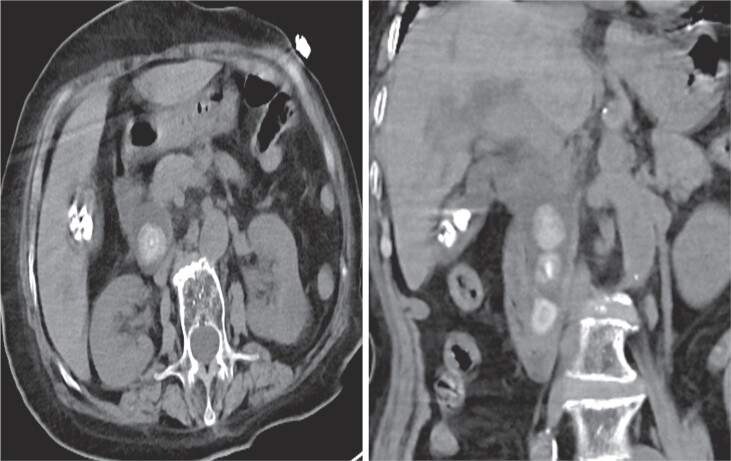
Admission whole-abdomen computed tomography (CT) showing
